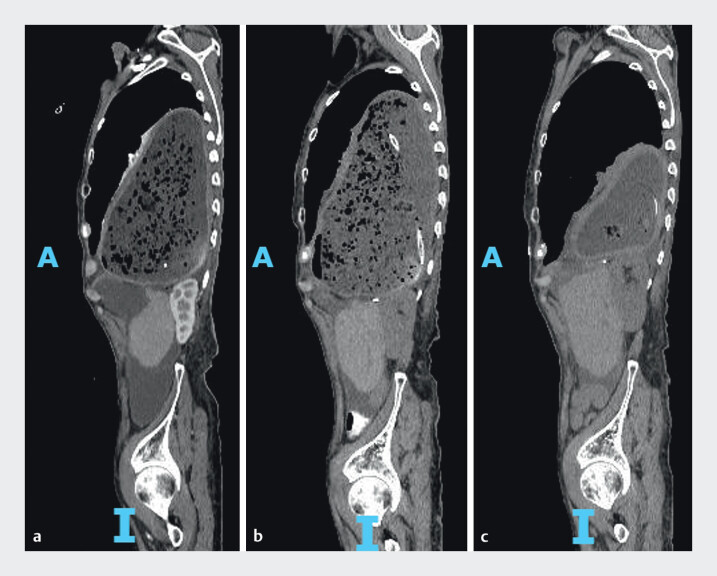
Computed tomography images obtained at the same sagittal plane level.
